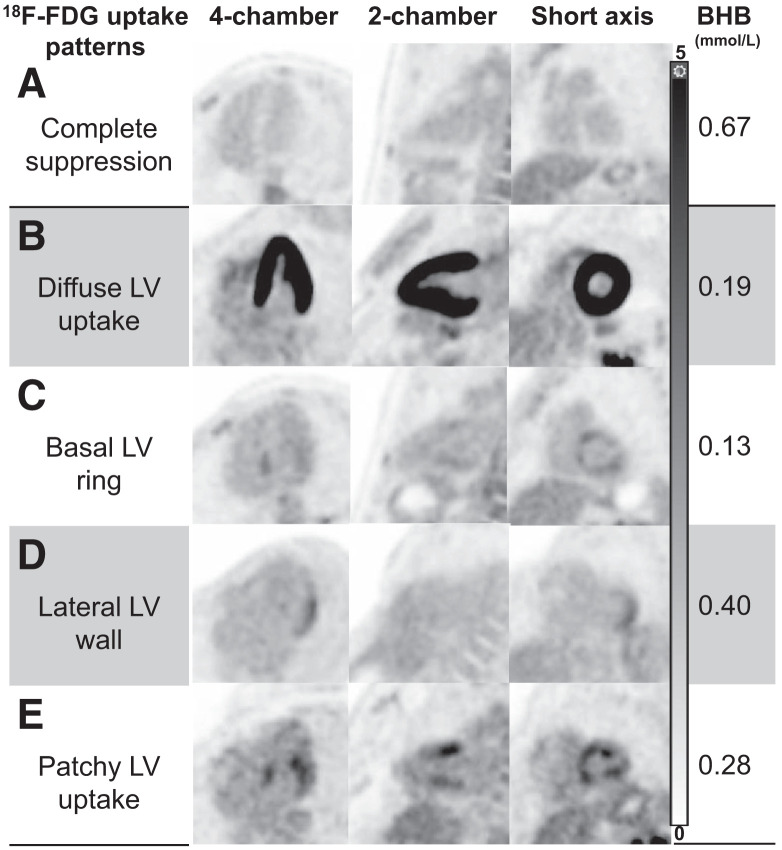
Myocardial ^18^F-FDG uptake patterns in healthy volunteers. Representative images (displayed using same window width 0–5) of most common myocardial ^18^F-FDG uptake patterns encountered in our healthy cohort, with their corresponding BHB levels. Patterns C–E can be potentially mistaken as myocardial inflammation; however, accompanying BHB levels should raise concern for incomplete suppression. LV = left ventricular.
